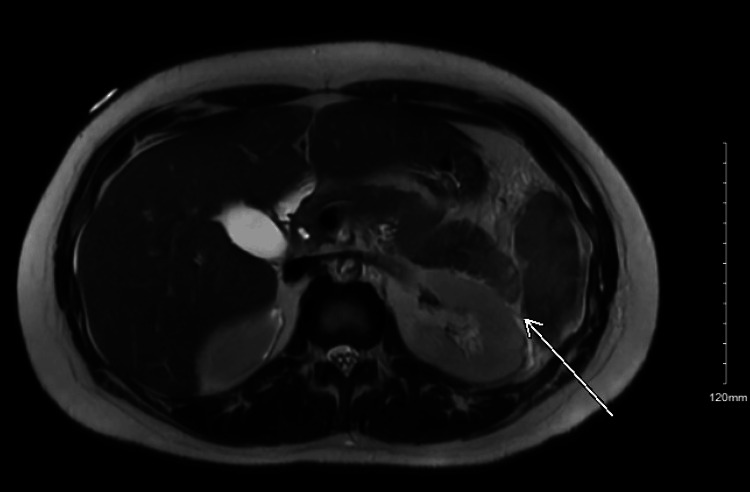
MRI confirming focal acute interstitial edematous pancreatitis.
